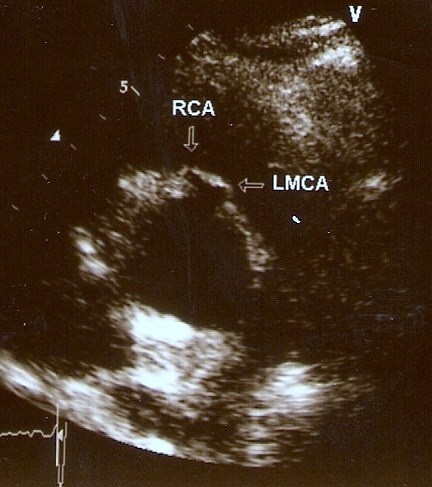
Common origin of both RCA and LMS from the right coronary cusp in echocardiogram.
